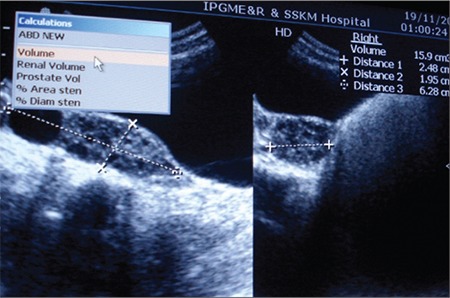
Ultrasonography of the abdomen showing bilateral polycystic ovaries with presence of a dominant cyst in the right ovary
